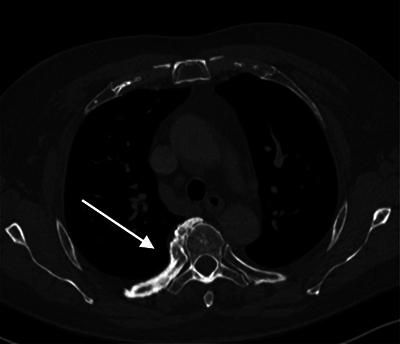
CT thorax axial images in bone window.
